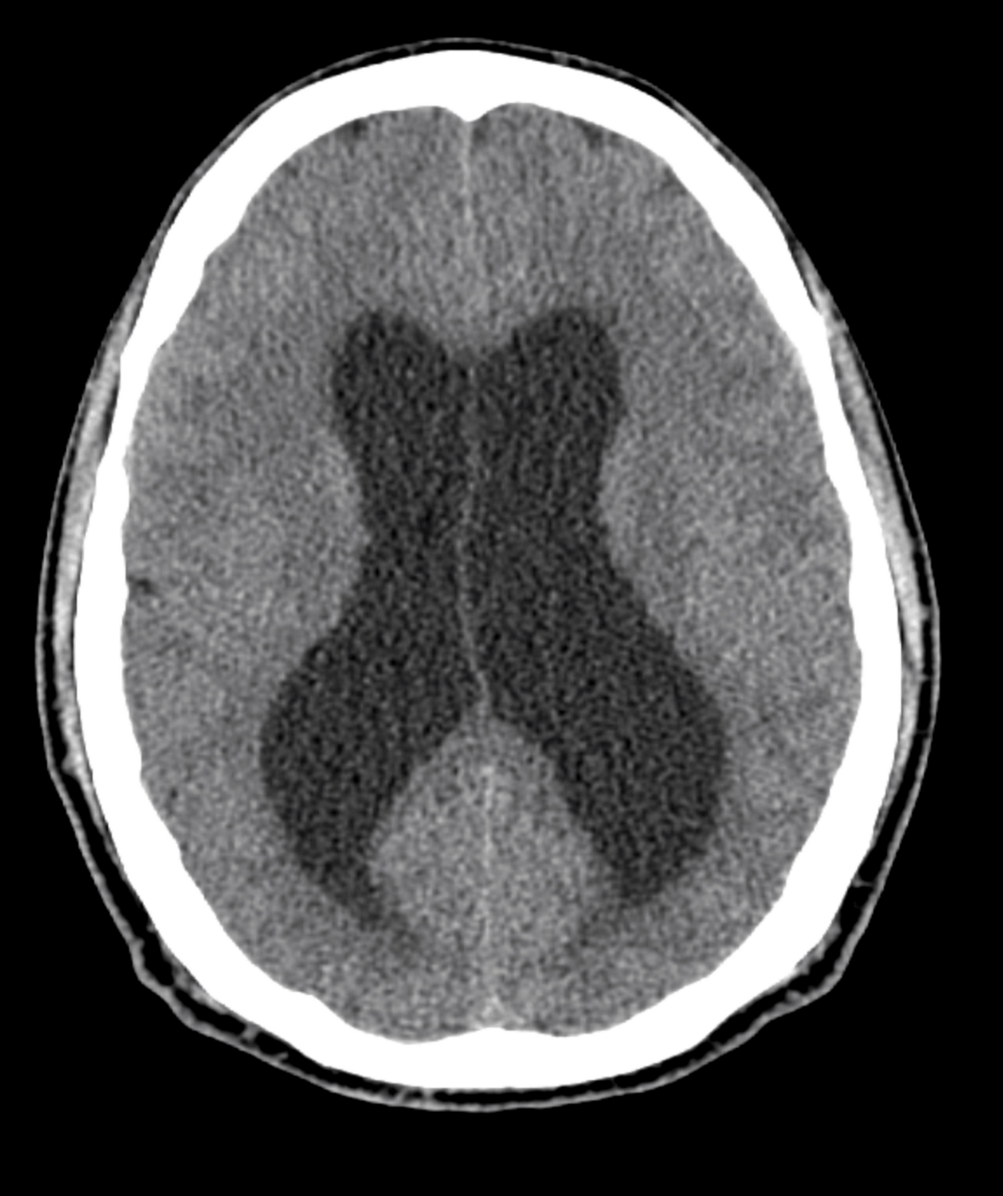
Axial head CT demonstrating hydrocephalus with enlargement of the lateral ventricles.
